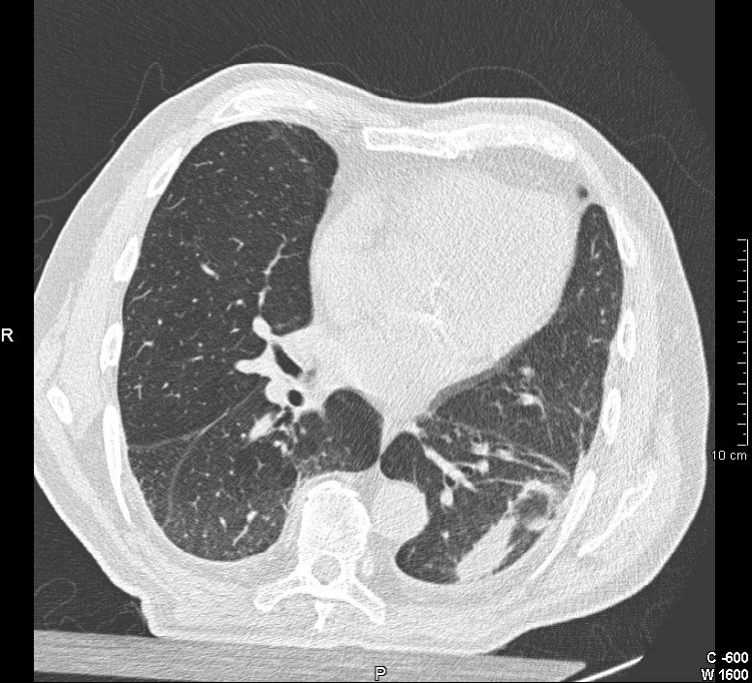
CT scan of the thorax demonstrating "folded lung" or Blesovsky's syndrome in association with diffuse pleural thickening.
